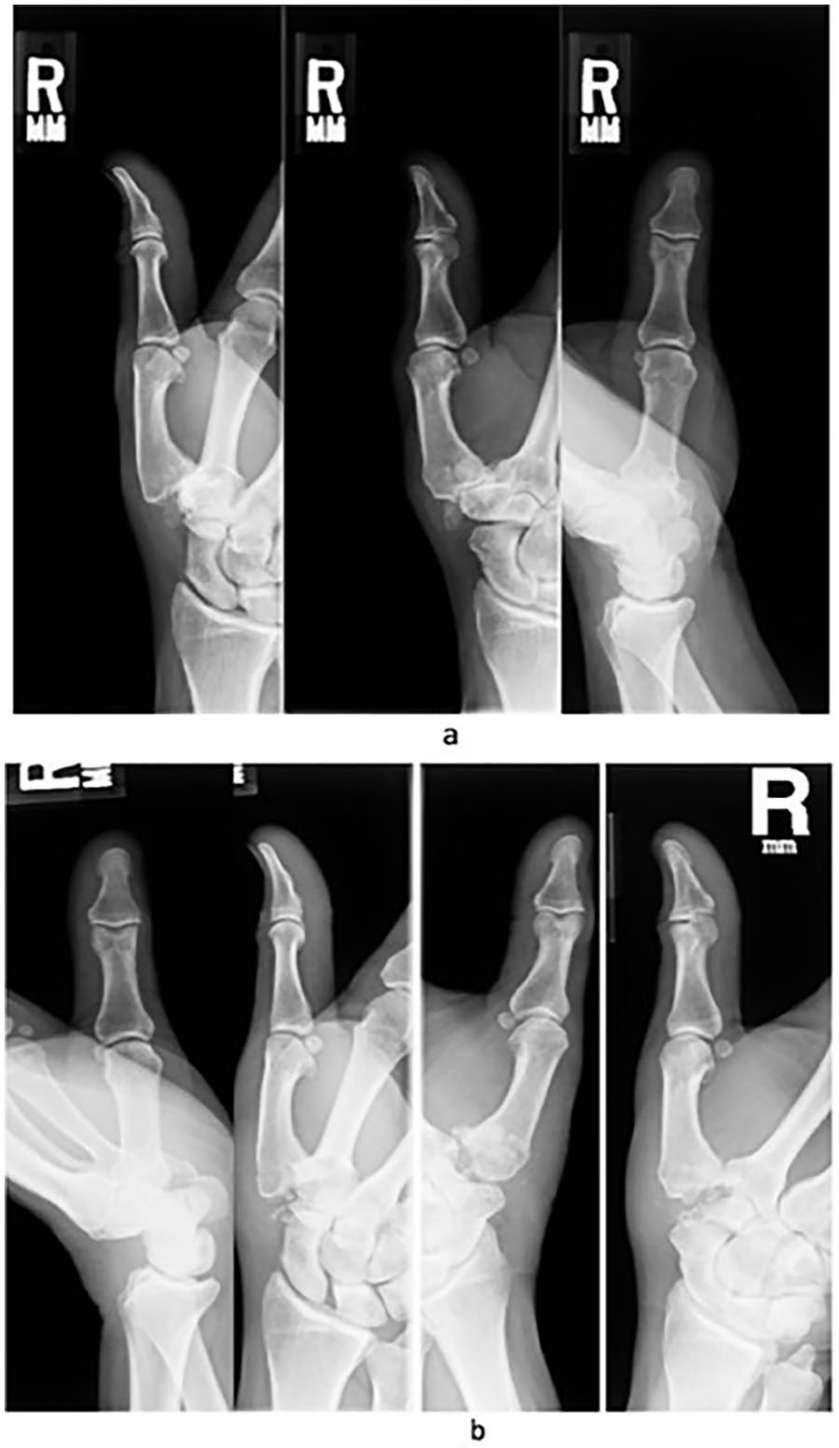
Radiographs of a patient with carpometacarpal arthroplasty: (a) preoperative and (b) postoperative.
